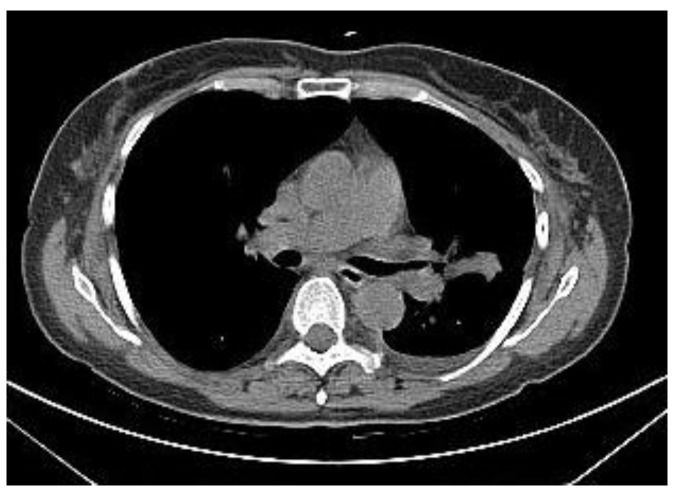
CT scan identification of a hilar lymph node as a sentinel lymph node.
